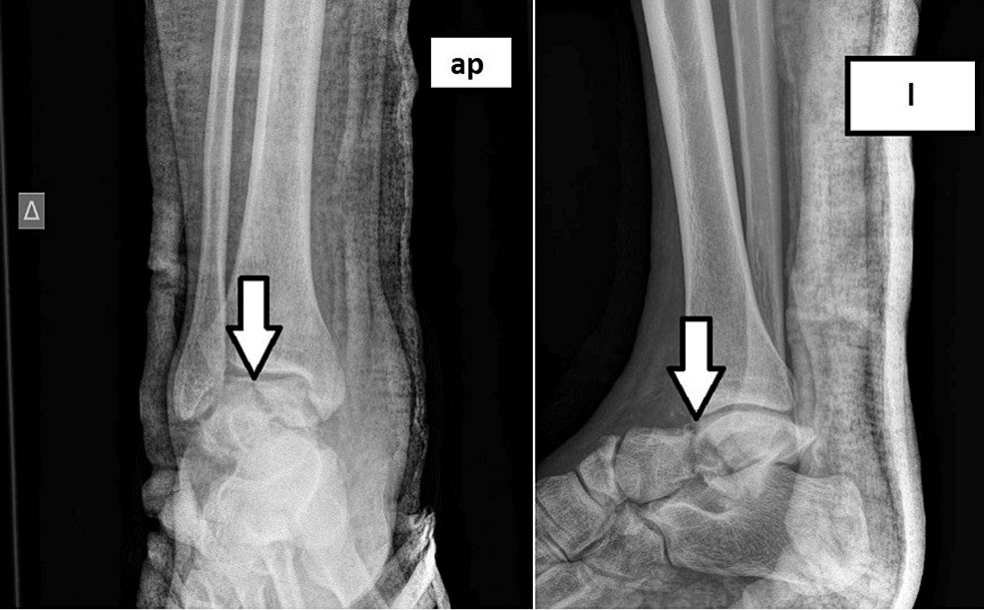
Initial radiographic evaluation depicts a talar neck fracture - Anteroposterior (AP) and Lateral (L) View
